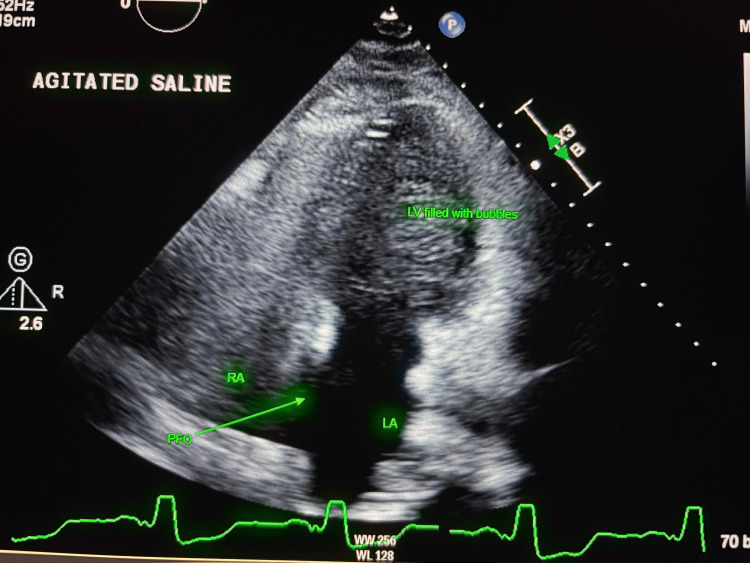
Apical four-chamber transthoracic echocardiographic view obtained during agitated saline (“bubble”) study demonstrating the LV filled completely with bubbles, indicating fairly large PFO. RA and LA are labeled for orientation. Appearance is consistent with evaluation for right-to-left interatrial shunting. LA: left atrium, LV: left ventricle, PFO: patent foramen ovale.
